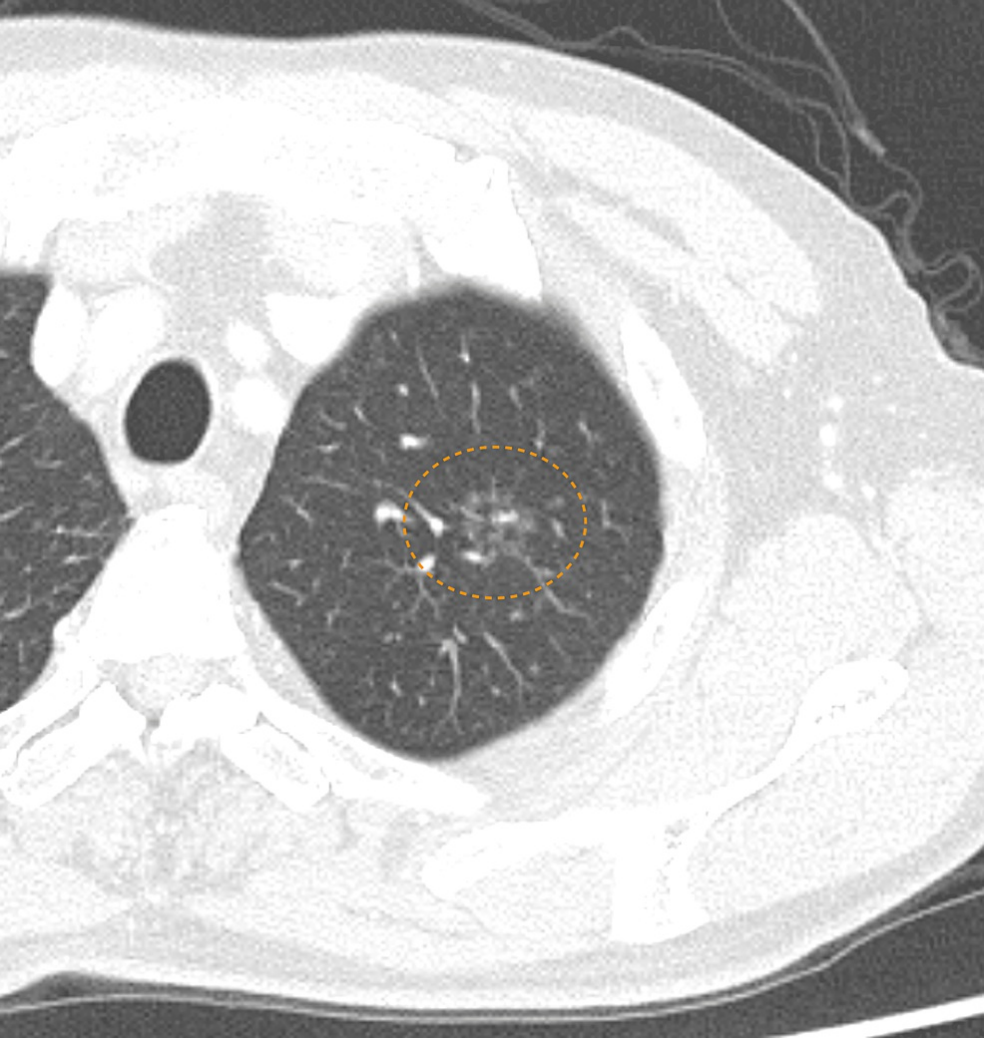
Preoperative chest CT Preoperative chest CT reveals a partially solid, ground-glass nodule containing a solid component in the left apicoposterior segments (S^1+2^).
